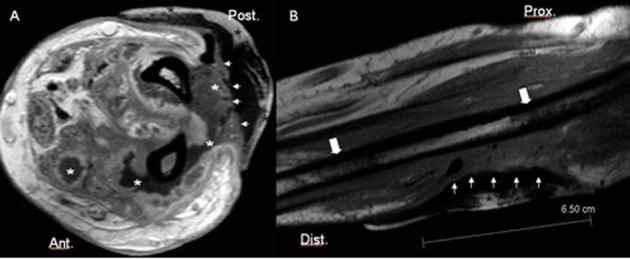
Axial T1-weighted images after injection of Gadolinium (A) and sagittal T1-weighted images before contrast injection (B). Multiple confluent avascular areas of soft tissues necrosis (white asterisks) of the posterior and the anterior compartment of the forearm are observed. The large area of skin necrosis (white arrowheads) is covered with a bandage (black asterisk). Some areas of bone marrow oedema of the radius are seen near the abnormal changes of soft tissue (large white arrows).
